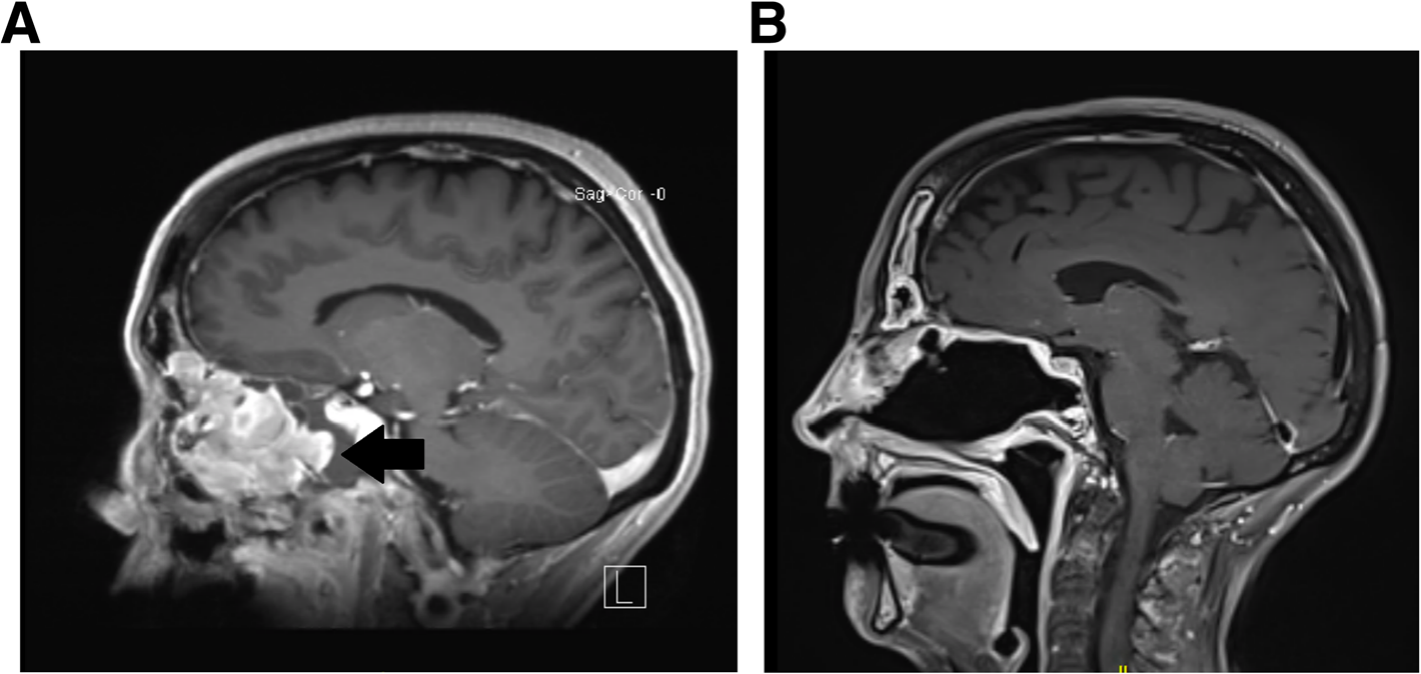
MRI Imaging of the Olfactory Neuroblastoma. Pre (a) and post (b) operative sagittal brain MRIs from our proband. The arrow on the left image indicates the olfactory neuroblastoma (neuroesthioblastoma)
